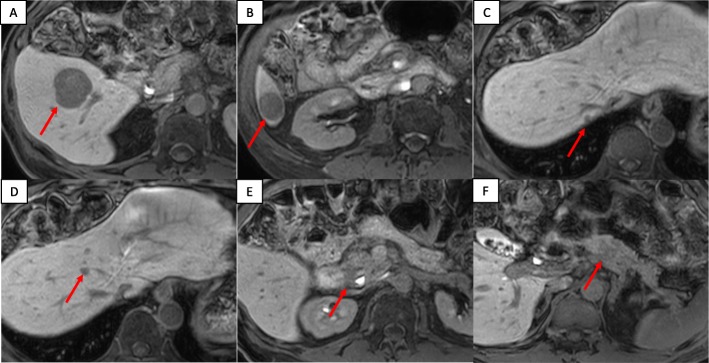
MRI image of liver and pancreatic tumors. An abdominal MRI showed multiple liver and pancreatic tumors. Each arrow shows the lesion. a S5 tumor. b S6 tumor. c S7 tumor. d S8 tumor. e Pancreatic head tumor. f Pancreatic body tumor
